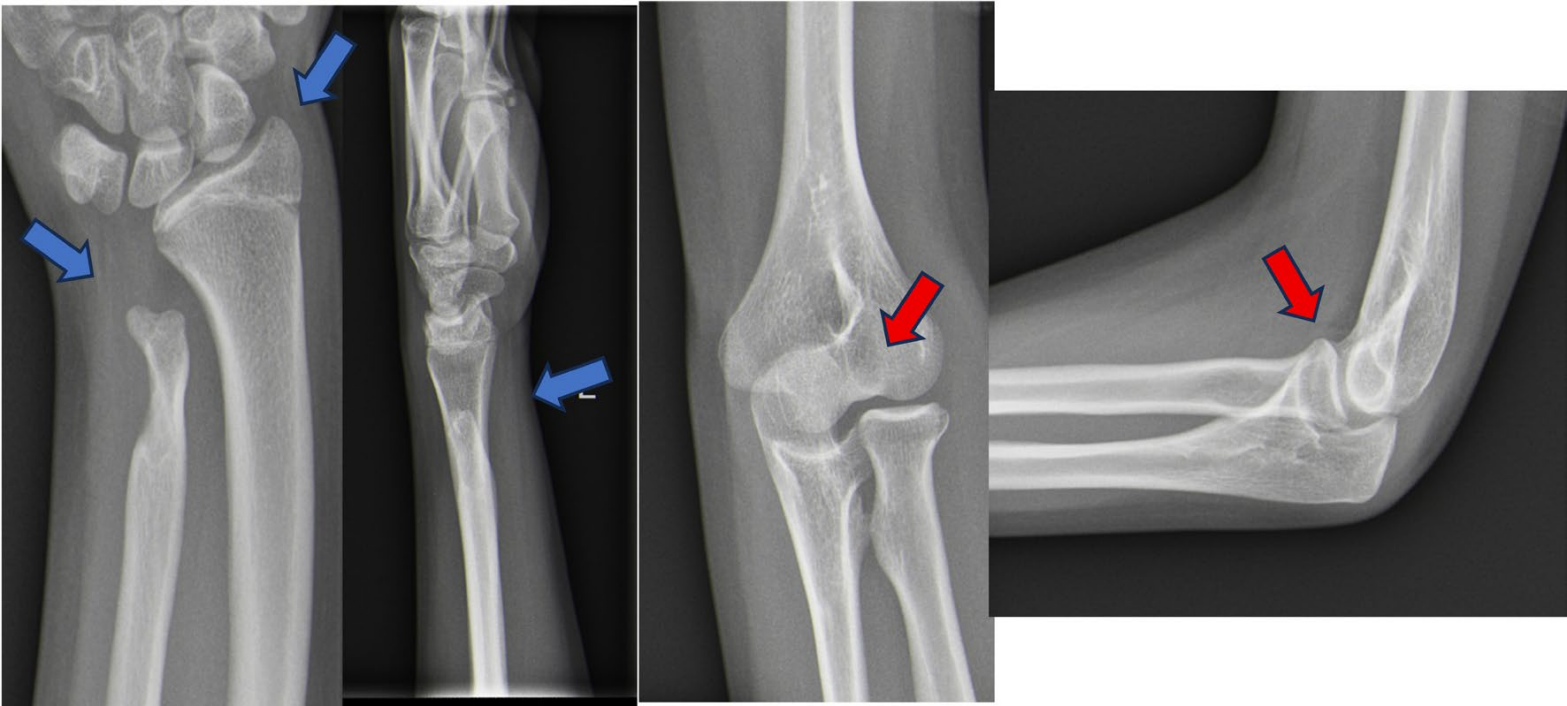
Anteroposterior and lateral radiographs of the wrist demonstrating a Madelung‐type deformity with increased volar tilt of the distal radius and negative ulnar variance (red arrows). The elbow shows the subluxation of the proximal radioulnar joint (blue arrows).
